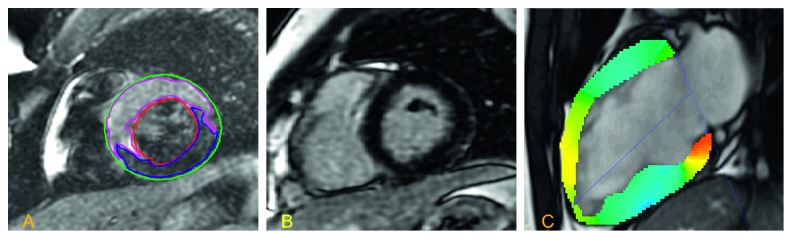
Cardiac MRI showing left ventricular dysfunction with edema and strain abnormalities predominantly in the apical segments suggesting an LAD territory insult. Edema in mid-anterior wall without any late gadolinium enhancement. (a) Increased image intensity of edema-based imaging in the anterior segments. (b) No corresponding late gadolinium enhancement is noted. (c) Movie attached. Still image showing hypokinesis of the anteroapical segments.
